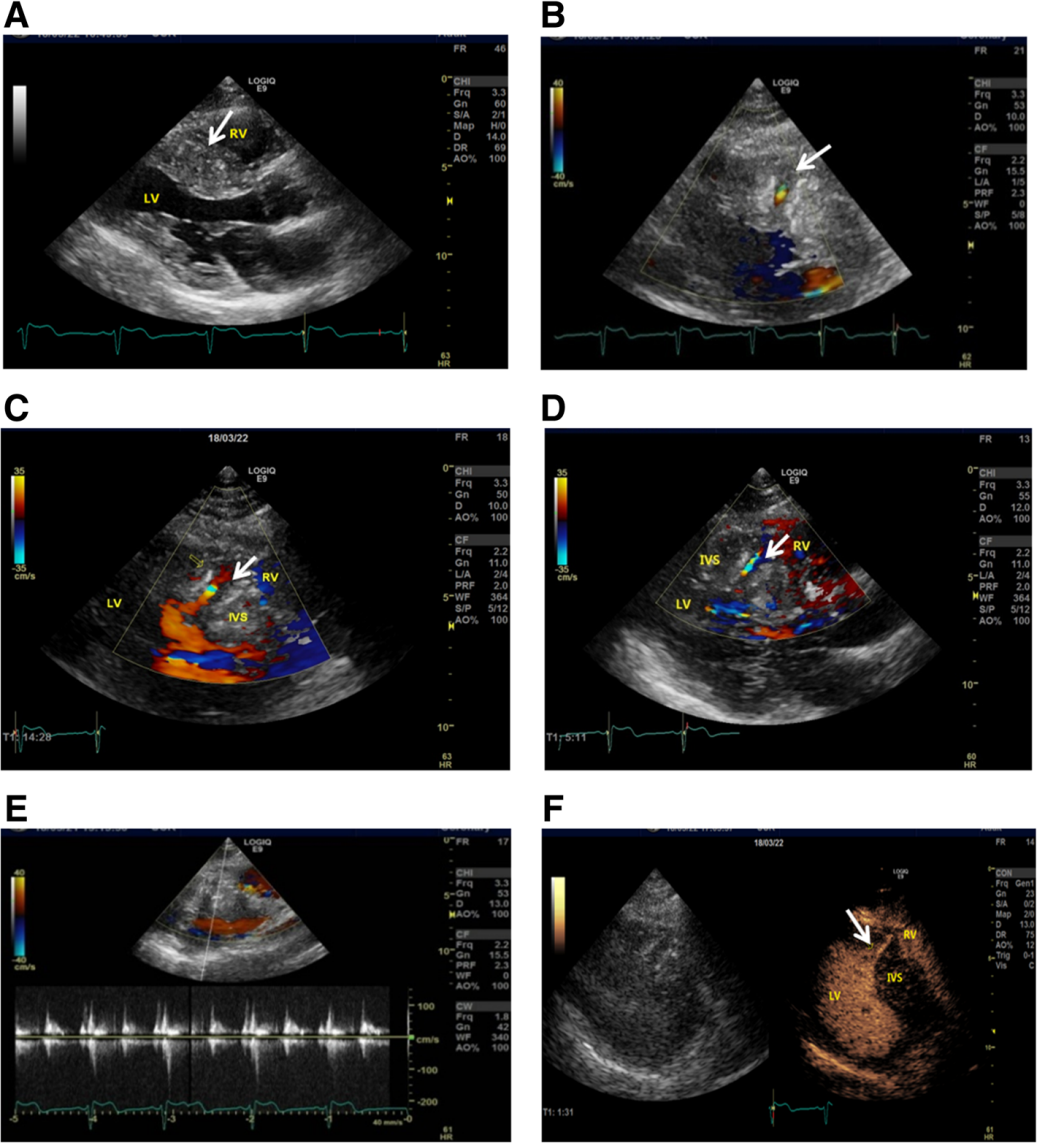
Representative images of hypertrophic interventricular septum, muscular VSD and anomalous blood flow signals by TTE. a Hypertrophic interventricular septum (white arrow). b Non-standard parasternal view showing small muscular defect with the echo enhancement of the broken end and blood flow in systolic phase (white arrow). c Systolic blood flow (white arrow) from left ventricle into the interventricular myocardium. d Systolic blood flow (white arrow) from interventricular myocardium into the left ventricle. e Image of a peak flow speed of 1.1 to 1.3 m/s during the systolic phase. f Contrast-enhanced echocardiography showed small defects (white arrow) being interlinked with the left ventricular cavity, but not with the right ventricular cavity. LV: left ventricle; RV: right ventricle; IVS: interventricular septum
